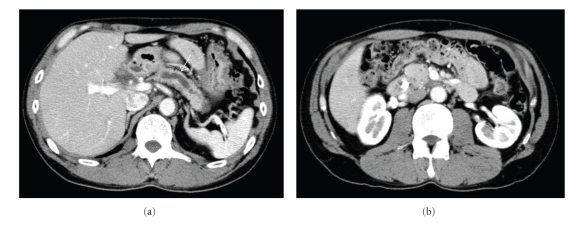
Enhanced CT image. A reduction in diameter of the PD (arrow) and the disappearance of the pseudocyst were demonstrated.
